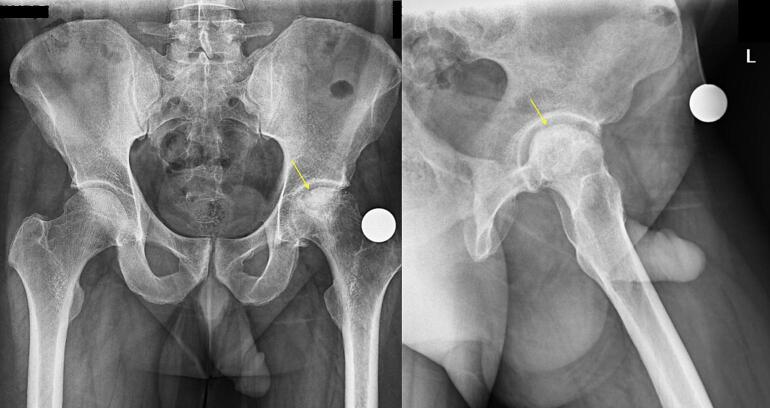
Pre-operative x-ray, left hip joint involvement. Left femoral head collapse along with osteonecrosis. A, AP view; B, Lateral view.
